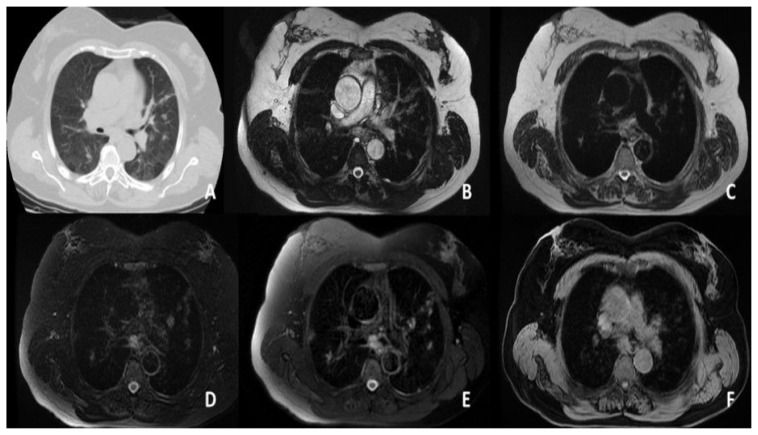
Patches of the GG and consolidation in both lungs, with more on the left. A. CT. B. FIESTA sequence. C. SSFSE sequence. D. T2 FS sequence. E. T2 PROPELLER sequence. F. T1 LAVA-F sequence.
